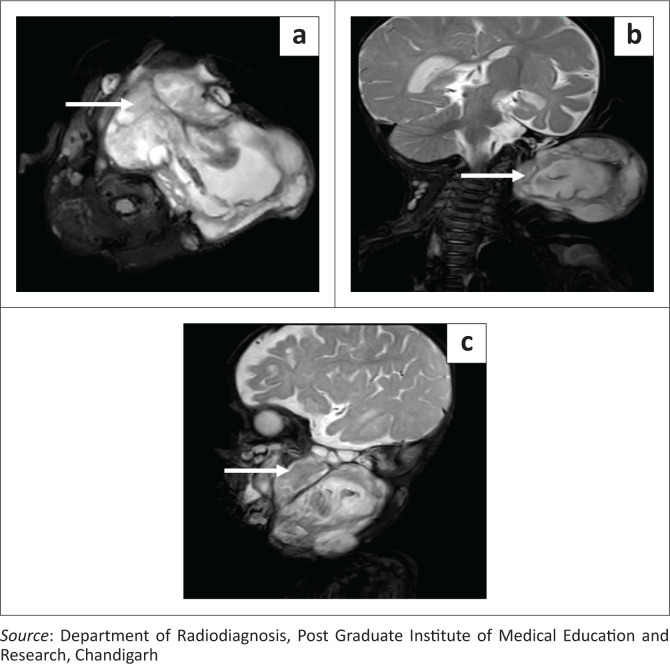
Axial (a), coronal (b), and sagittal (c) T2-weighted MR images demonstrate a large, trans-spatial, solid-cystic lesion involving the left cervical region. The cystic components are T2 hyperintense, while the eccentric solid component (arrows) demonstrates T2 iso to hypointense signal similar to grey matter. The lesion extends medially into the parapharyngeal space and laterally into the parotid and submandibular spaces, resulting in focal outward bulging of the overlying skin contour.
